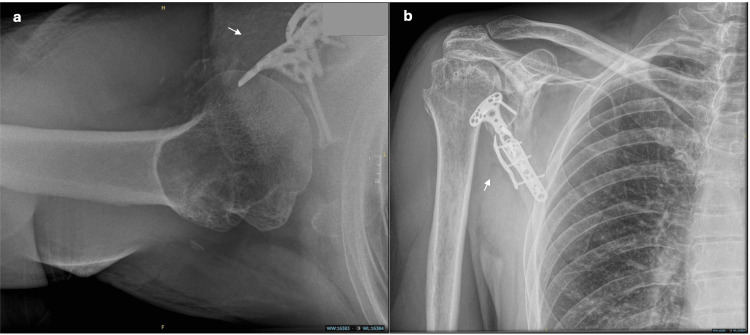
Three-week post-operative radiographs. a. Axial shoulder X-ray. b. Anterior-posterior shoulder X-ray. Figures 9a-9b show the distal radius plate remained in a stable position, and fracture reduction was well-maintained three weeks post-operatively (white arrows).
